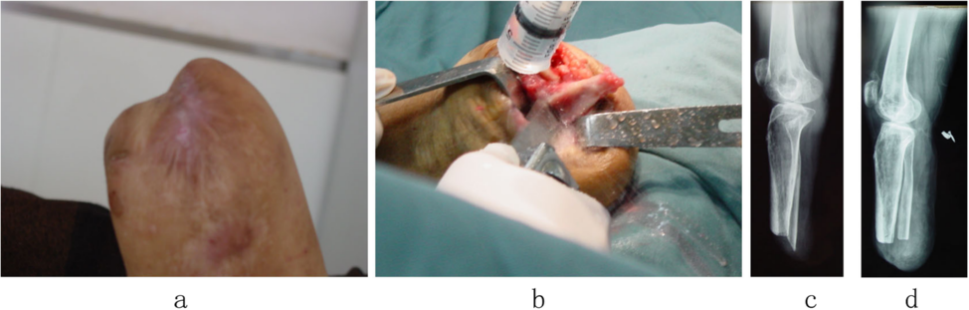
Scar, longer fibula, stump pain influence weight-bearing. Bone protrusion and scar on weight-bearing impeded the fitting of prosthesis (a); dissection of the longer fibula and scar,trim the bone end smoothly and then suture with myoplasty (b); a sharp fibular end showed on the X-ray before operation (c); the fibula residual end was smooth and soft tissue was cylindrical on the X-ray after operation (d).
